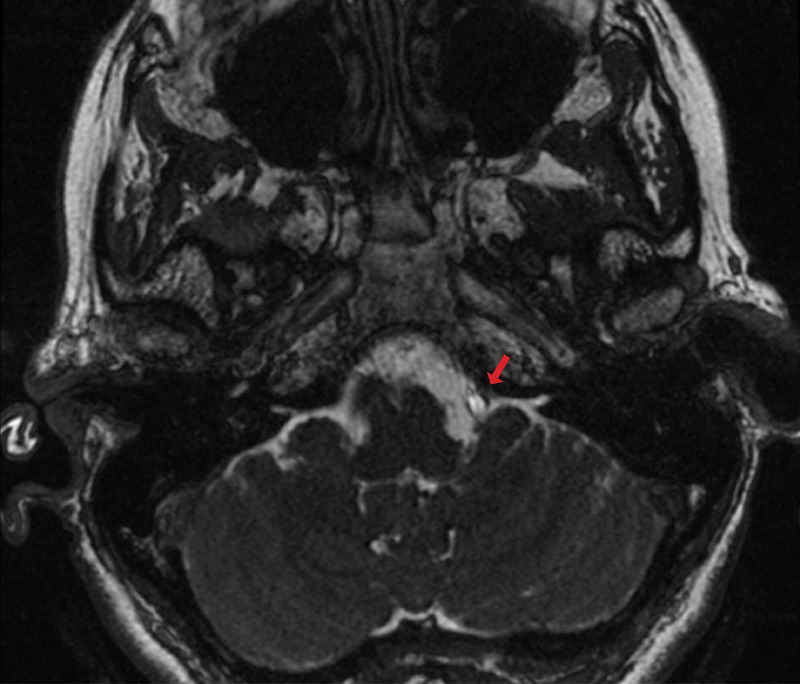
Preoperative MRI demonstrating a left dominant vertebral artery (arrow) causing compression on cranial nerves IX and X
